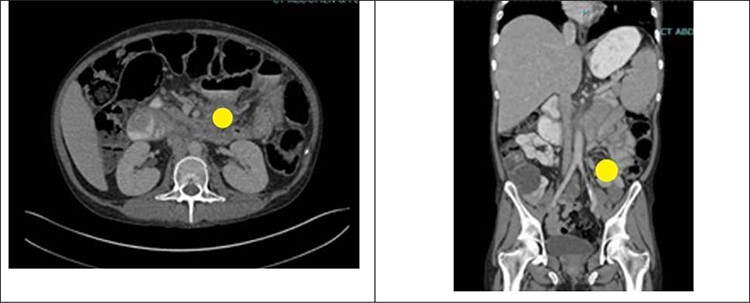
CT abdomen and pelvis with IV contrast showing an ill-defined mass at the root of the mesentery measuring at least 5.2 × 5.1 cm (yellow circle). This was hypothesized to represent conglomerate lymphadenopathy, a potential neoplasm, such as mesothelioma or lymphoma, or metastatic disease. There was also a large volume of abdominal ascites and mildly dilated loops of small bowel within the left abdomen which likely represented a partial obstruction or ileus.
